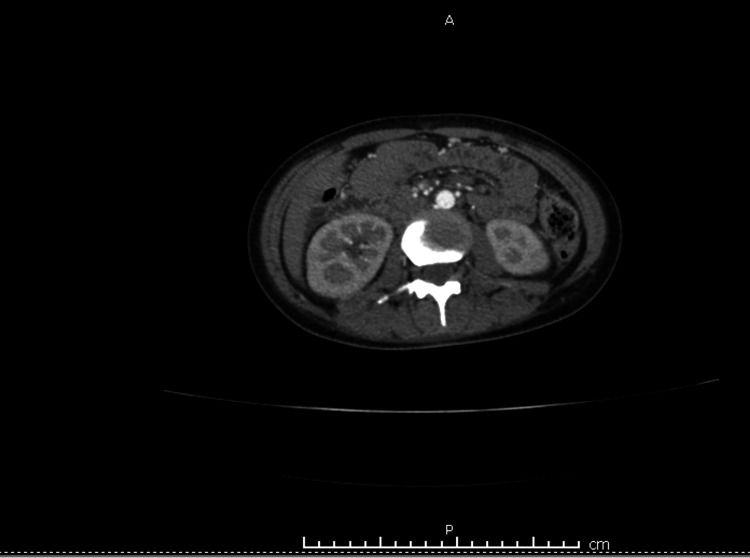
Abdominal CT showing kidney infarcts due to septic emboli
